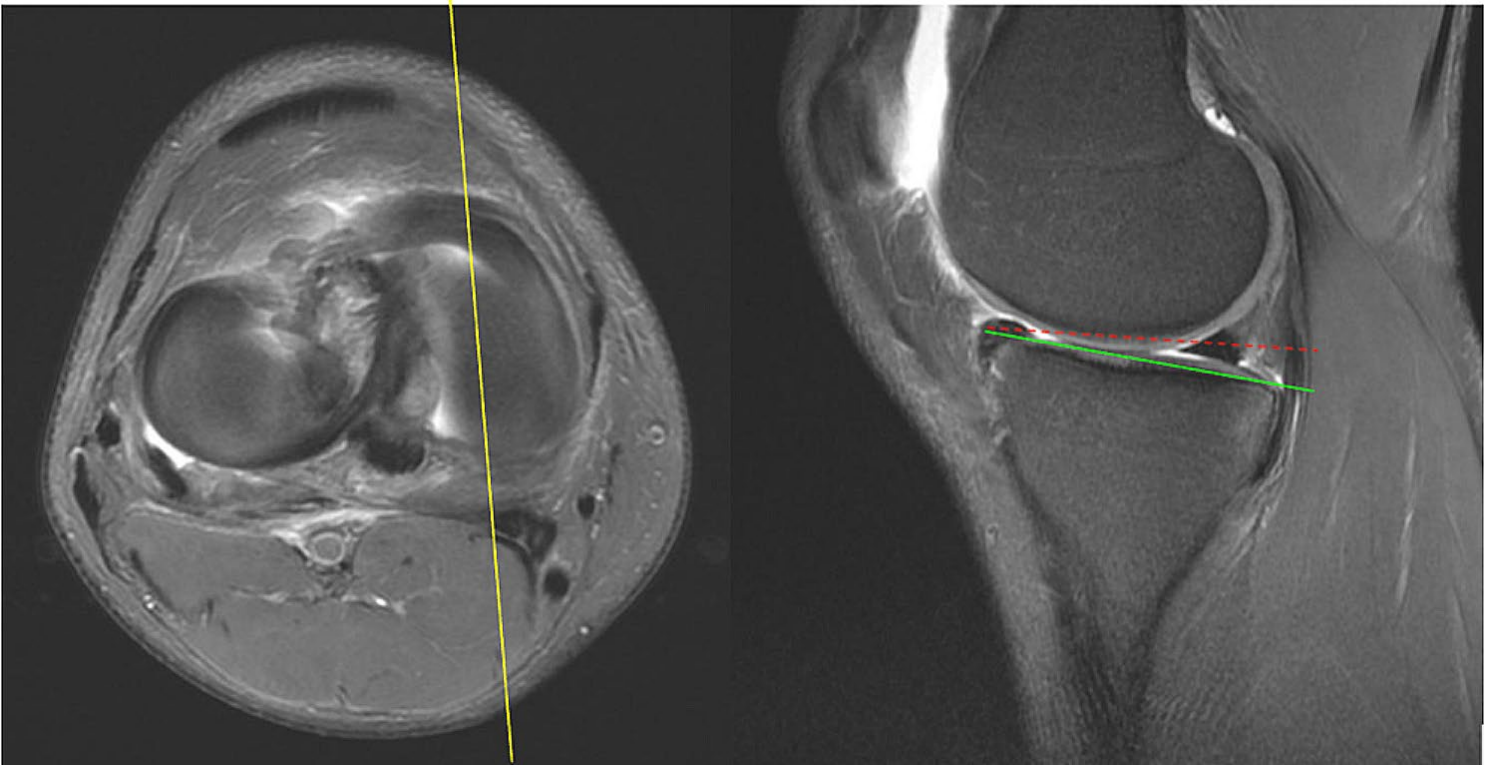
Measuring the medial tibial slope: The reference line is adjusted to the center of the medial tibial plateau. Connect the peak points of the anterior and posterior aspects of the plateau on the Sagittal MRI (green line). The angle between the green line and the dotted red line is defined as the medial tibial slope
